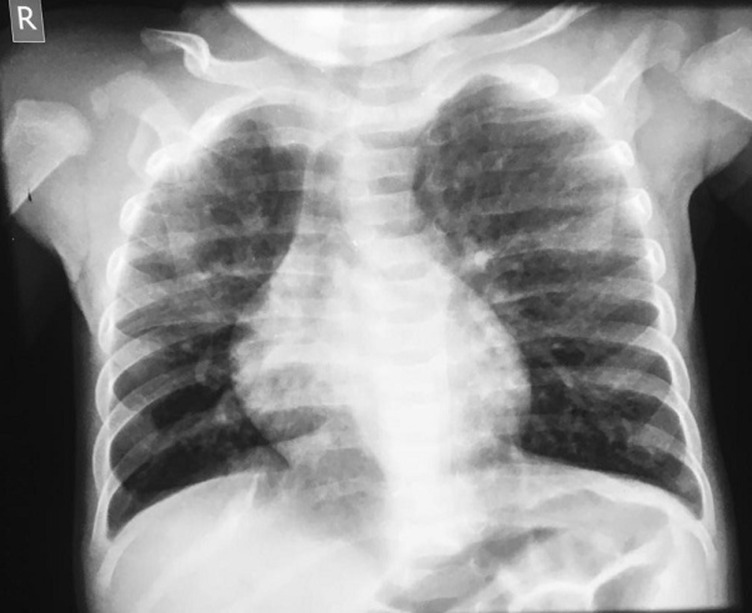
chest X-ray findings in a 2 months old ex-premature female child with *T. ptyseos* septicaemia
